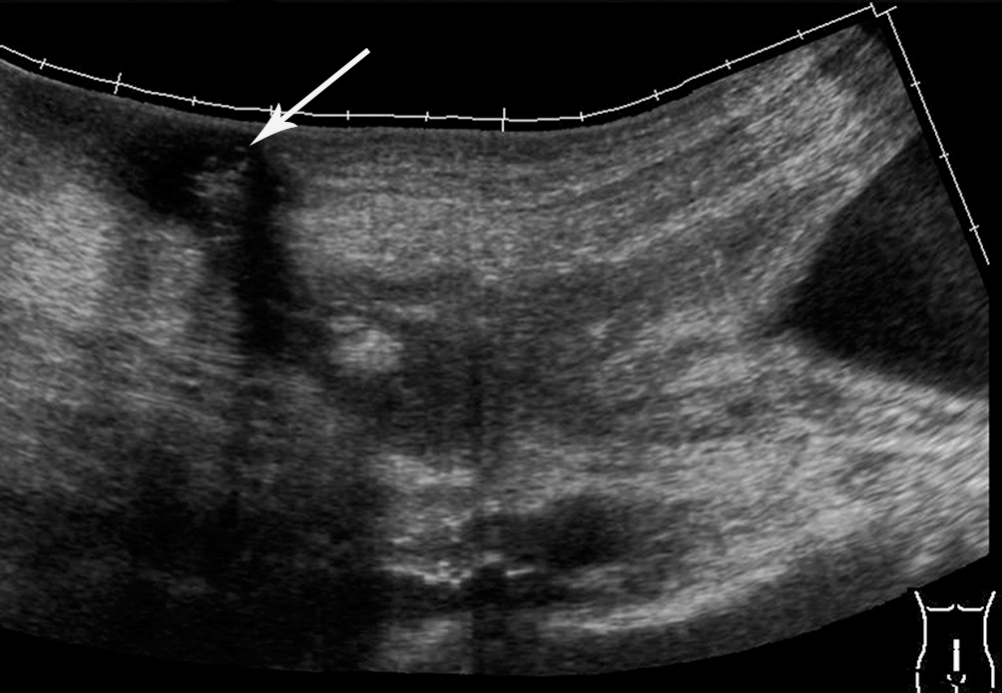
Panoramic image of the abdominal ultrasound showing patent urachus, with presence of gas (arrow).
